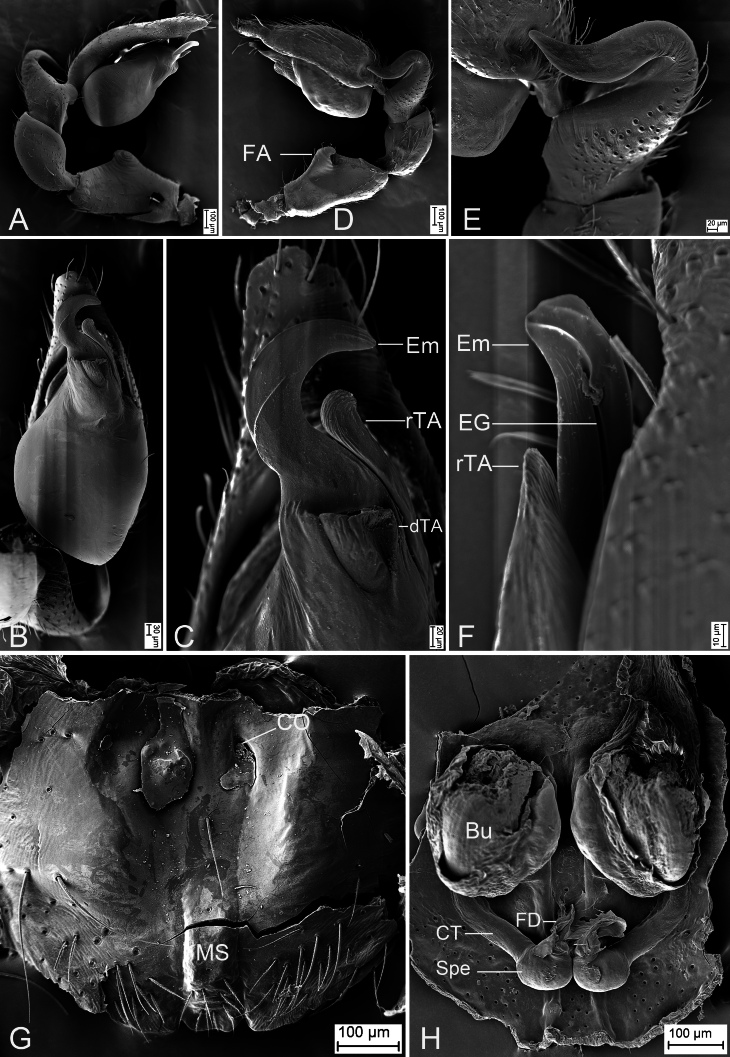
Otaciliatongboshan* sp. nov., male palp and female epigyne, paratype. A Palp, prolateral view; B Same, ventral view; C Same, detail of Em, rTA and dTA, ventral view; D Same, retrolateral view; E Same, detail of RTA, retrolateral view; F Same, detail of Em, EG and rTA, retrolateral view; G Epigyne, ventral view; H Same, dorsal view. Abbreviations: Bu − bursa, CO − copulatory opening, CT − connecting tube, dTA − distal tegular apophysis, EG – embolic groove, FA − femoral apophysis, FD − fertilisation ducts, MS − median septum, rTA − retrolateral tegular apophysis, RTA − retrolateral tibial apophysis, Spe − spermathecae.
